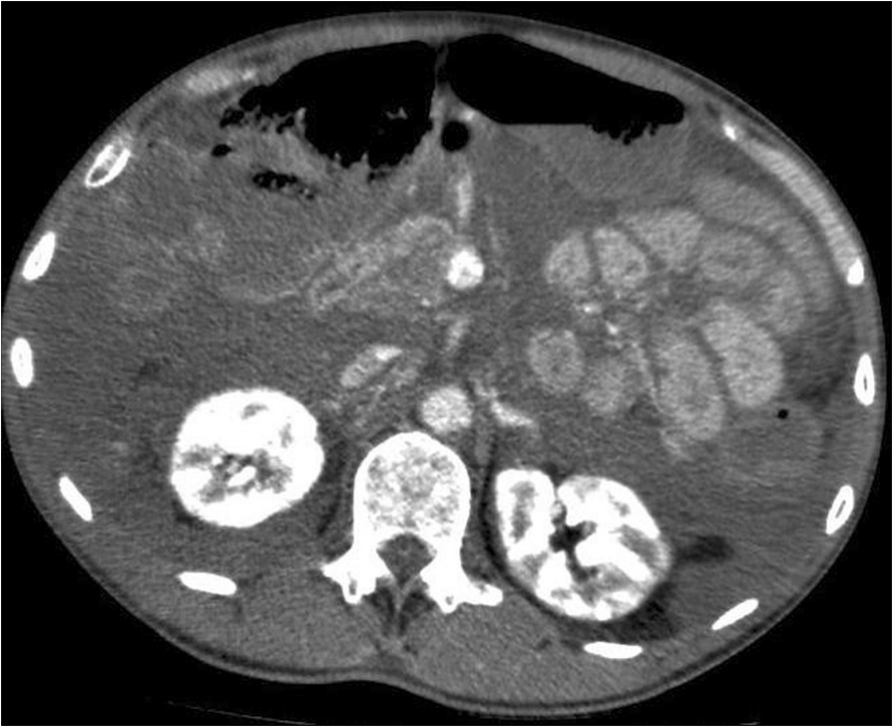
CT Abdomen of patient 1, here at kidney–level, showing the dense mucous masses initially interpreted as pseudomyxoma peritonei filling the abdominal cavity, making the individual organs difficult to see. On this second CT-scan from day 2, the masses are increasingly dense, now measuring around 50 HU. There are no obvious signs of bowel ischemia.
